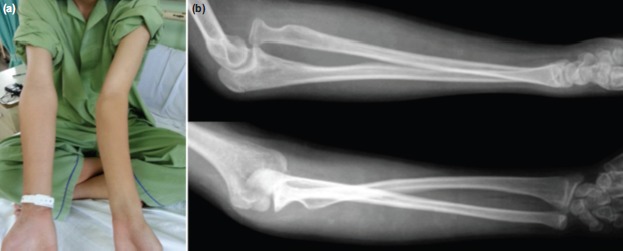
(a) Pre-operative clinical picture with cubital valgus of 45 degree and (b) Pre-operative radiograph with radial head dislocated anteriorly.
